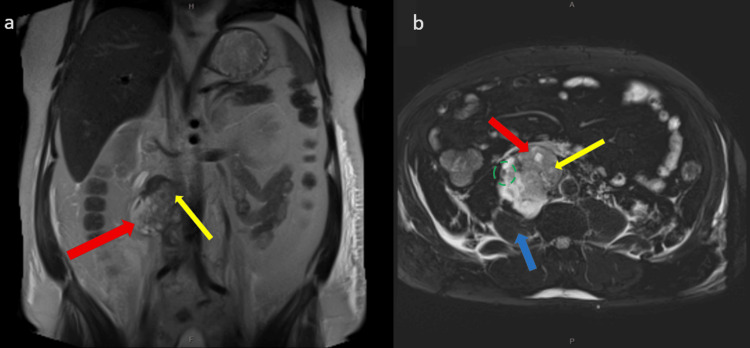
MRI T2 phase pre-operatively MRI: magnetic resonance imaging; IVC: inferior vena cava (a) Coronal slice demonstrating a right-sided malignant tumour (red arrow) with involvement of the third part of the duodenum (yellow arrow). (b) Axial slice demonstrating a lobulated mass (red arrow) invading the IVC lumen (yellow arrow) with involvement of right ureter (green dotted oval), and right psoas (blue arrow)
